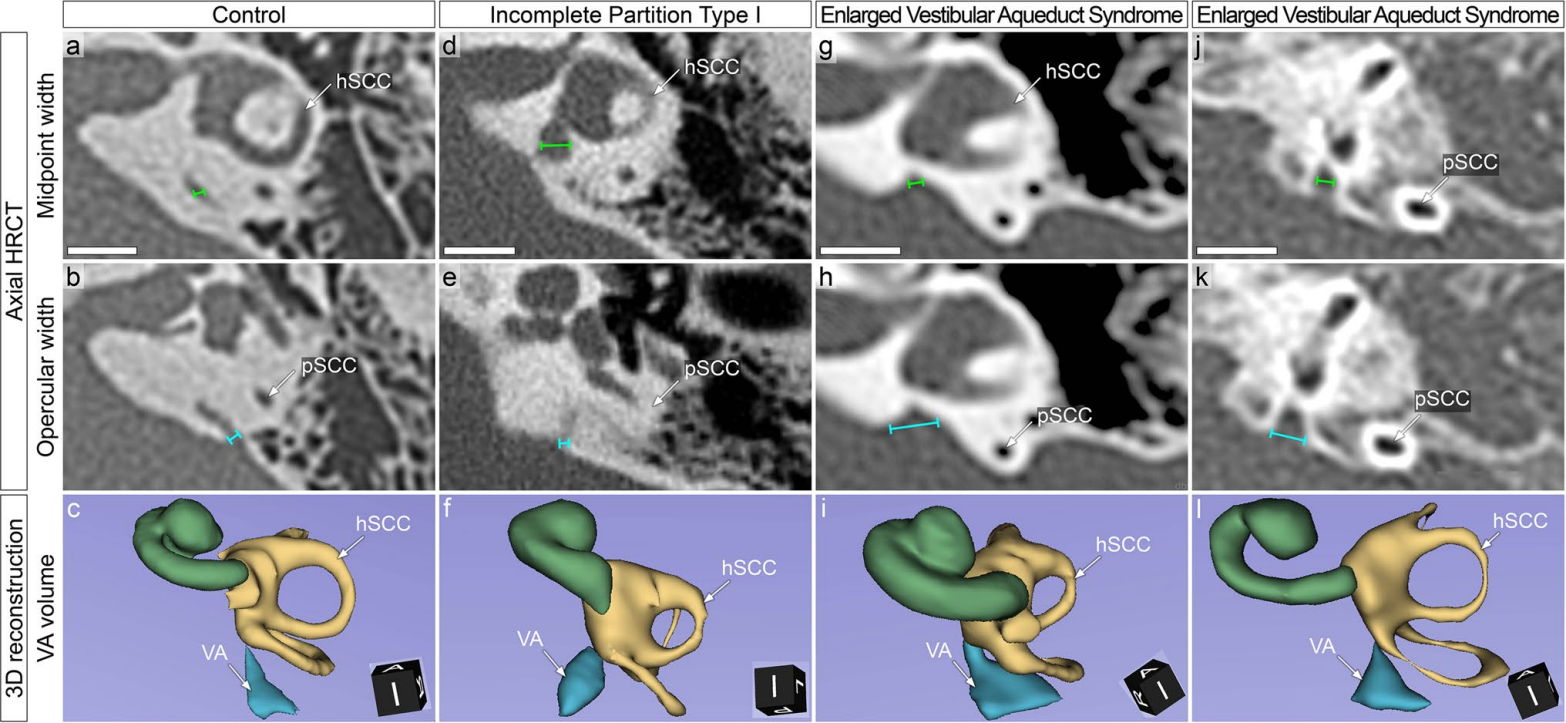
Exemplary vestibular aqueduct (VA) shapes in a control and three cases of inner ear malformation (IEM). a–c 2D-measurements of the VA_midpoint_ (a) and the VA_operculum_ (b) in the axial high-resolution computed tomography (HRCT) as well as three-dimensional (3D) reconstruction of the bony labyrinth (c) in a normal control. d–f Case 1, incomplete partition type I: two-dimensional (2D) measurements of the VA_midpoint_ (d) and VA_operculum_ (e) in the axial HRCT as well as a 3D reconstruction (f). The VA is centrally dilated with narrowing toward the operculum and the vestibule. g–i Case 2, enlarged vestibular aqueduct syndrome (EVAS): 2D measurements of the VA_midpoint_ (g) and VA_operculum_ (h) in the axial HRCT as well as 3D reconstruction of the bony labyrinth (i). j–l Case 3, EVAS: 2D-measurements of the VA_midpoint_ (j) and VA_operculum_ (k) in the axial HRCT as well as a 3D reconstruction of the bony labyrinth (l). In both case 2 and case 3, the VA is narrow toward the vestibule and shows a very broad opening toward the operculum. Both cases were classified as EVA according to the Cincinnati criteria (case 2: VA_midpoint_ = 1.0 mm, VA_operculum_ = 2.1 mm; case 3: VA_midpoint_ = 1.0 mm; VA_operculum_ = 1.9 mm). The volume of case 2 is 29.5 mm^3^. The volume of case 3 is 16.4 mm^3^. Green volume, cochlea; yellow volume: vestibular labyrinth; blue volume: VA. *hSCC* horizontal semicircular canal, *pSCC* posterior semicircular canal. Scale bars: 5 mm
